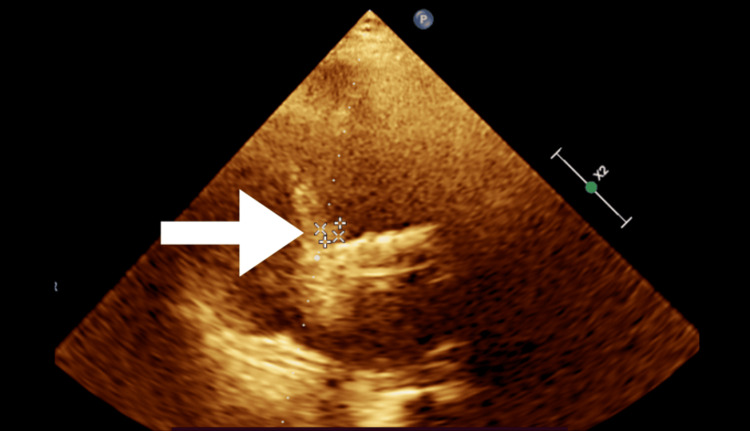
2D echocardiogram image The white arrow highlights the vegetation on the aortic valve.
